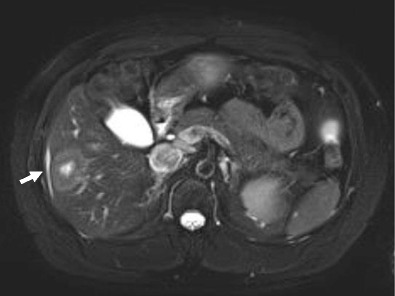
T2-weighted MRI demonstrates inflammatory pseudotumour as a target type lesion with markedly hyperintense centre and a mildly hyperintense margin in hepatic segment 5, with associated flattening of the overlying hepatic capsule
